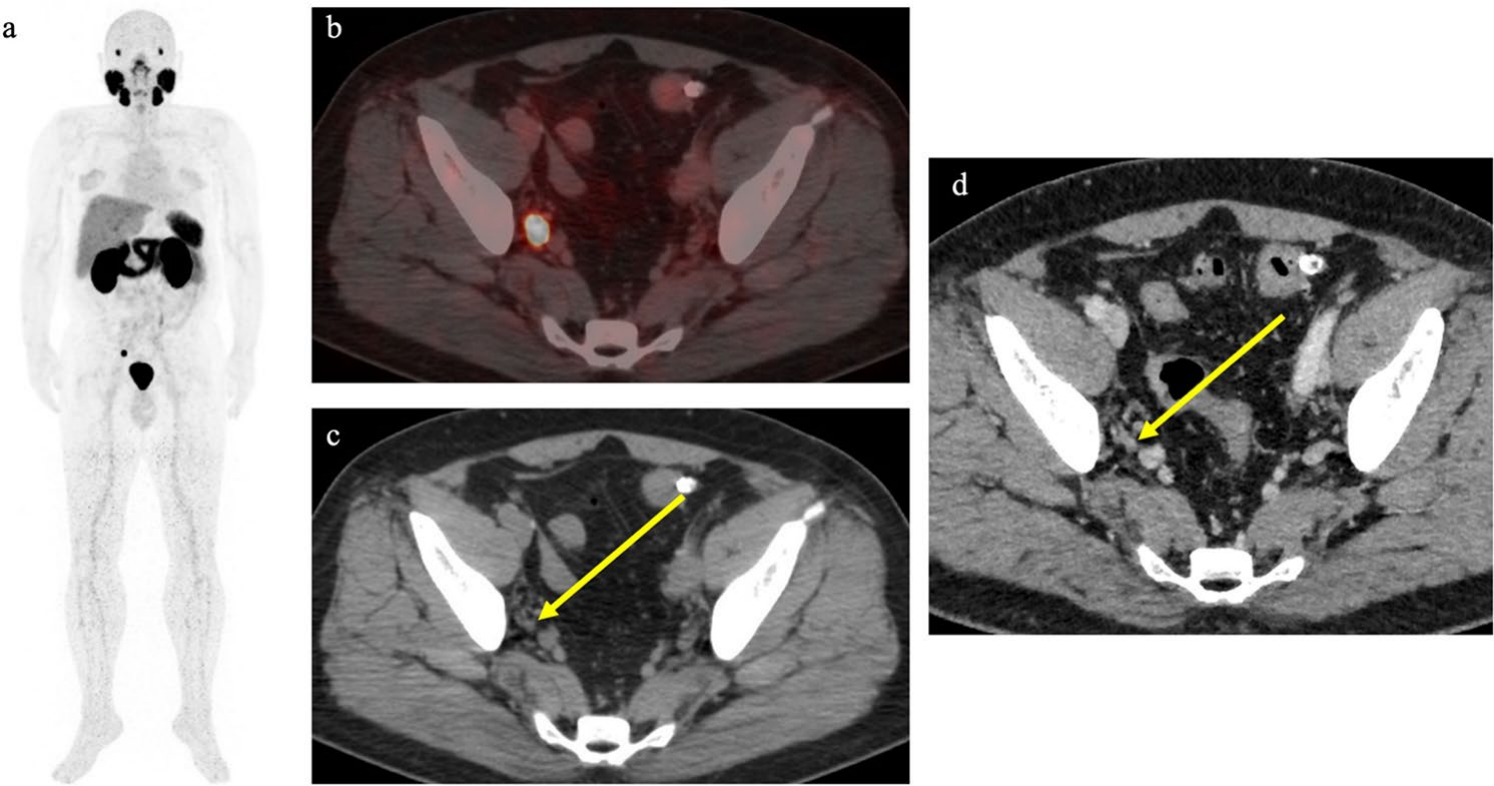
PET/CT using ^68^ Ga-PSMA-11 in a 65-year-old man with biochemical recurrence following total prostatectomy (pT3aN1, Gleason score 4 + 5). PSA level increased to 1.35 ng/ml. Focal uptake was observed in a 6-mm lymph node in the right obturator region (arrows), suggesting lymph-node metastasis. a Maximum intensity projection image. b Transaxial PET/CT fusion image. c Transaxial CT. d Transaxial contrast-enhanced CT
